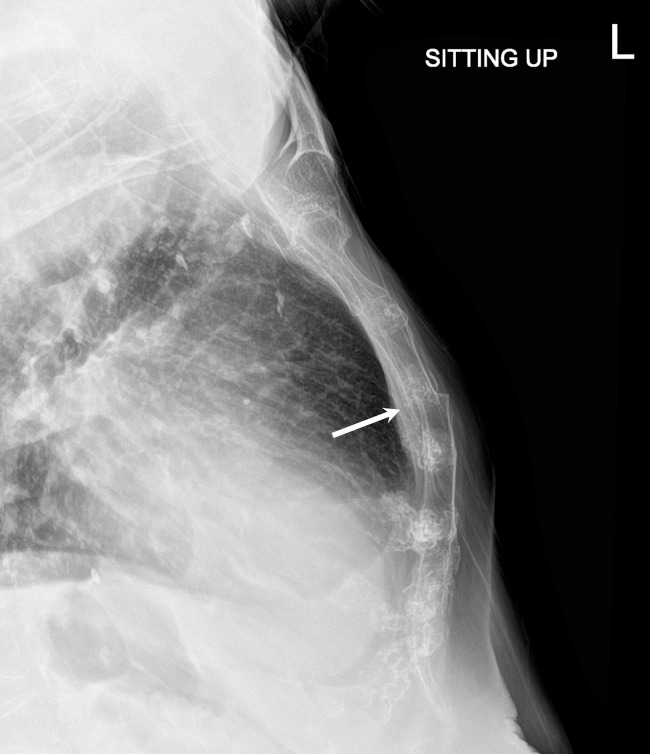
Follow-up lateral radiograph of the sternum shows increased displacement of the sternal fracture (arrow). This radiograph was obtained 2 weeks after the chest radiographs in Fig. 1.
